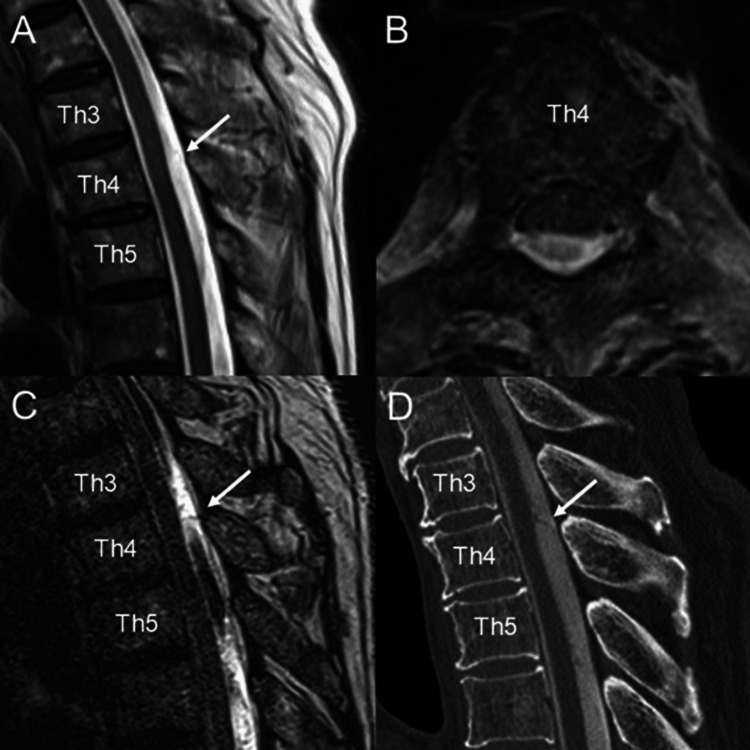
(A, B) Sagittal and axial T2-weighted images of the thoracic spine show mild dorsal compressive deflection of the spinal cord at the T4 level (white arrow). (C, D) A sagittal heavily T2-weighted image (C) and CT myelography (D) of the thoracic spine demonstrate a membrane-like structure dorsal to the spinal cord at the T4 level, suggestive of a SAW (white arrow). CT: computed tomography; SAW: spinal arachnoid web
